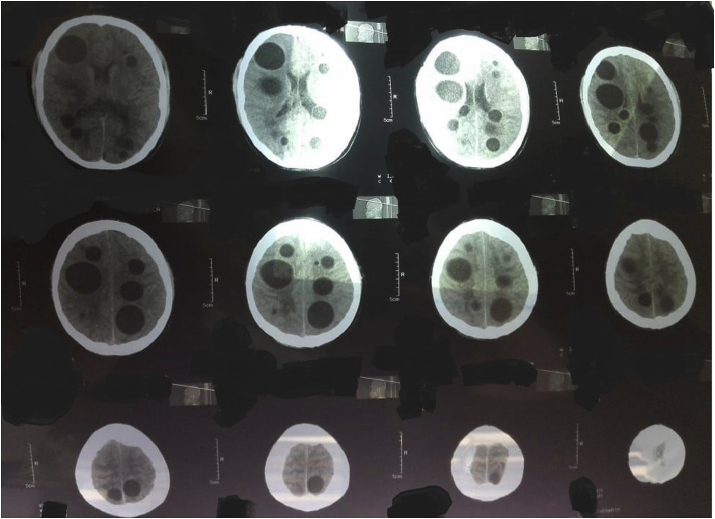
CT Scan brain 11 months postoperative open heart surgery.
